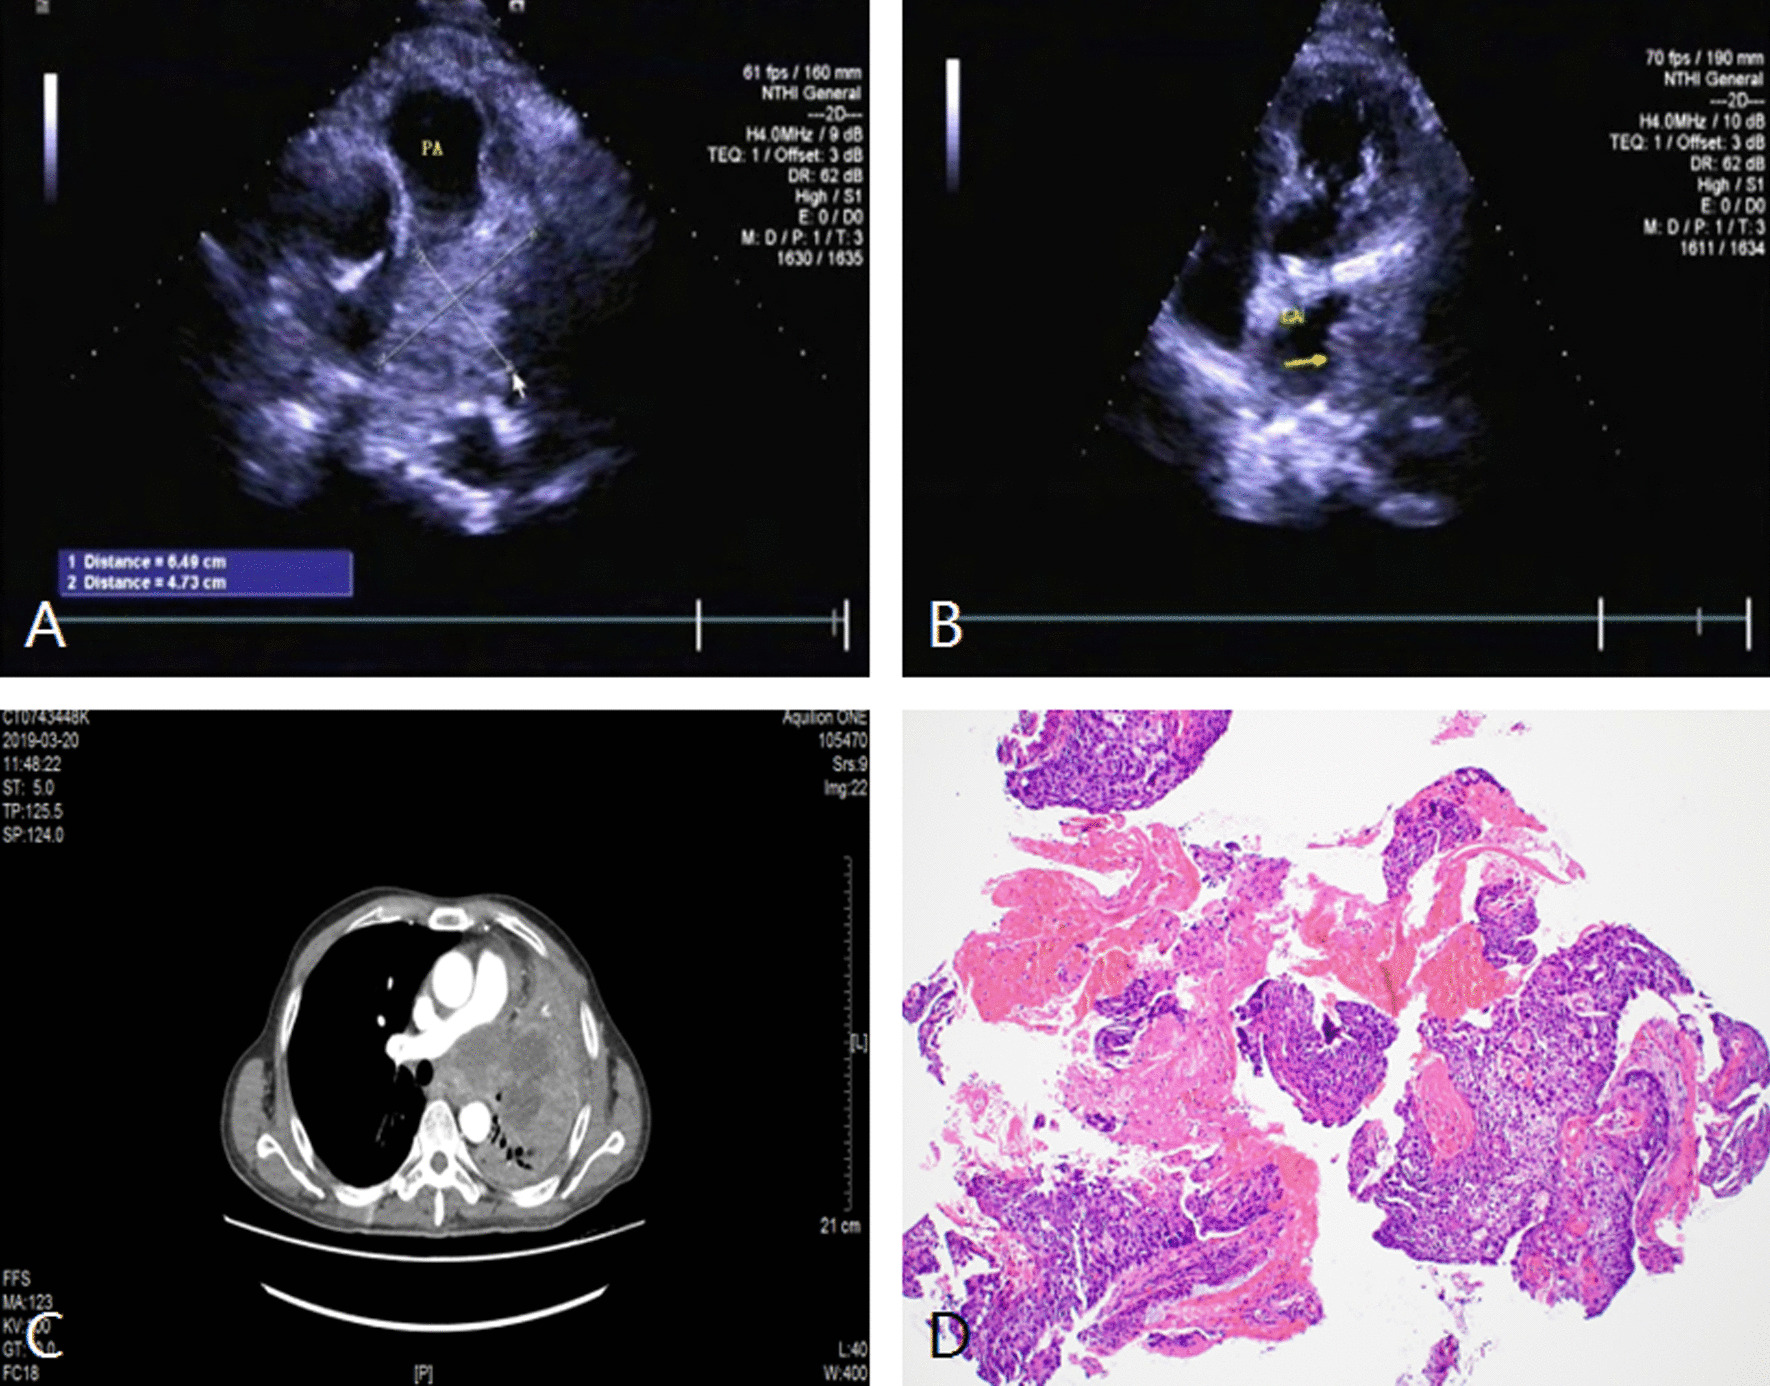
Left hilar lung cancer in a 51-year-old man. A and B TTE showed an irregularly shaped hyperechoic mass near the left hilum of the pulmonary artery bifurcation with left pulmonary artery and left pulmonary vein invasion. C Contrast-enhanced CT shows an irregularly shaped mass with mediastinal lymph node metastasis and obstructive atelectasis. D The pathologic diagnosis of this mass was moderately differentiated squamous cell carcinoma. TTE indicates transthoracic echocardiography; CT indicates computer tomograph; LA, left atrium; PA, pulmonary artery
